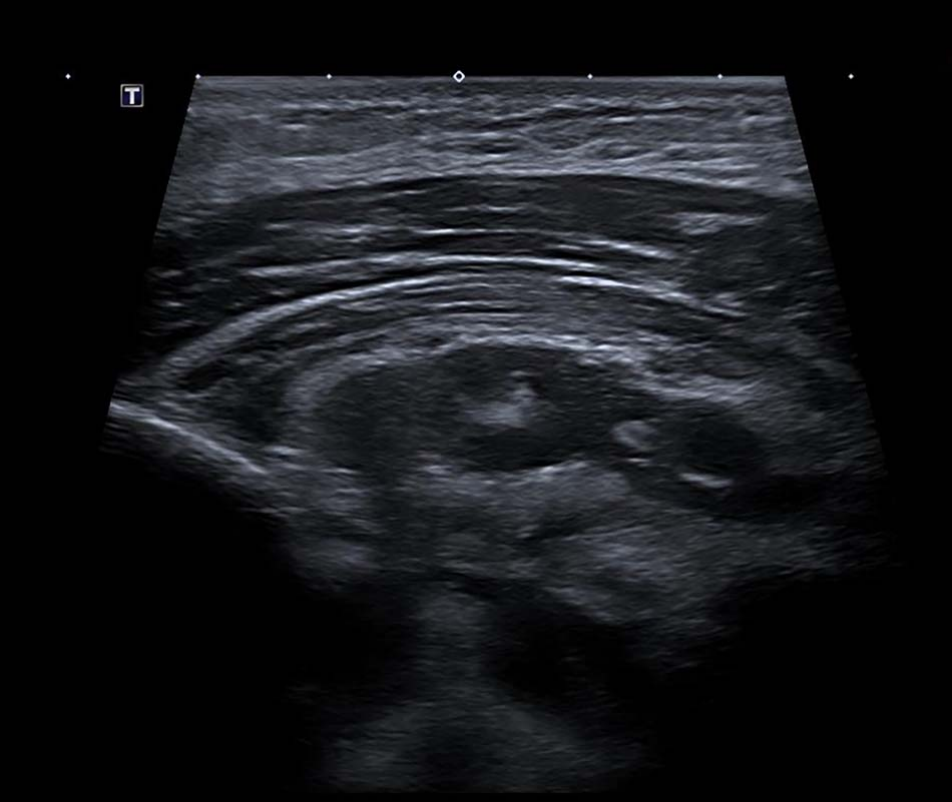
Ultrasound scan demonstrating a retropectoral abscess communicating with the mediastinum.
